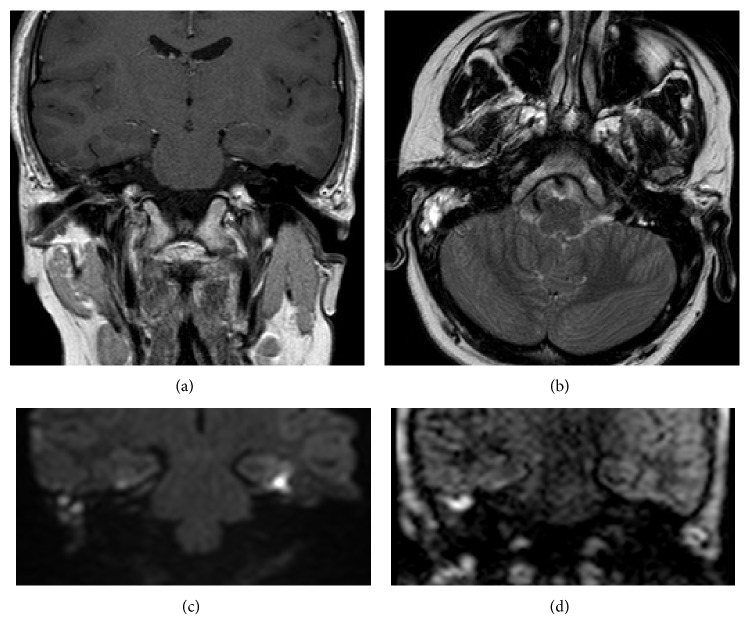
Images of primary cholesteatoma of the right middle ear cavity: (a) coronal SE T1w, (b) axial T2w TSE, (c) coronal SSh SE-EPI DWI (thickness 2 mm; TE 88.364 ms; TR 3774 ms; FOV 230 × 230 mm; number of average 4; *b* value: 0–800), and (d) coronal MSh TSE DWI (thickness 3 mm; TE 80.672 ms; TR 3000 ms; FOV 230 × 230 mm; number of average 2; *b* value: 0–1000).
